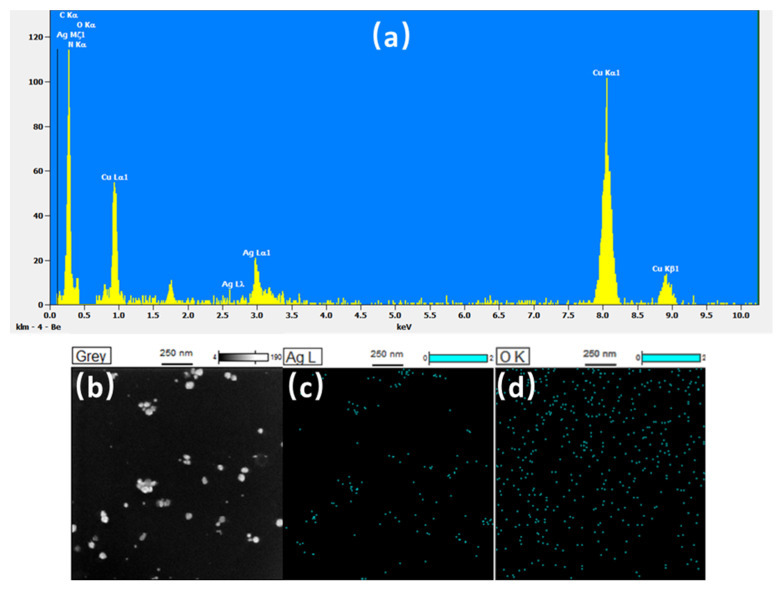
EDX spectra (a) and dark-field TEM image of Ag NPs (b) with the corresponding EDX map for silver (c) and oxygen (d) (ultrasonic frequency: 100 kHz, ultrasonic time: 120 min).
